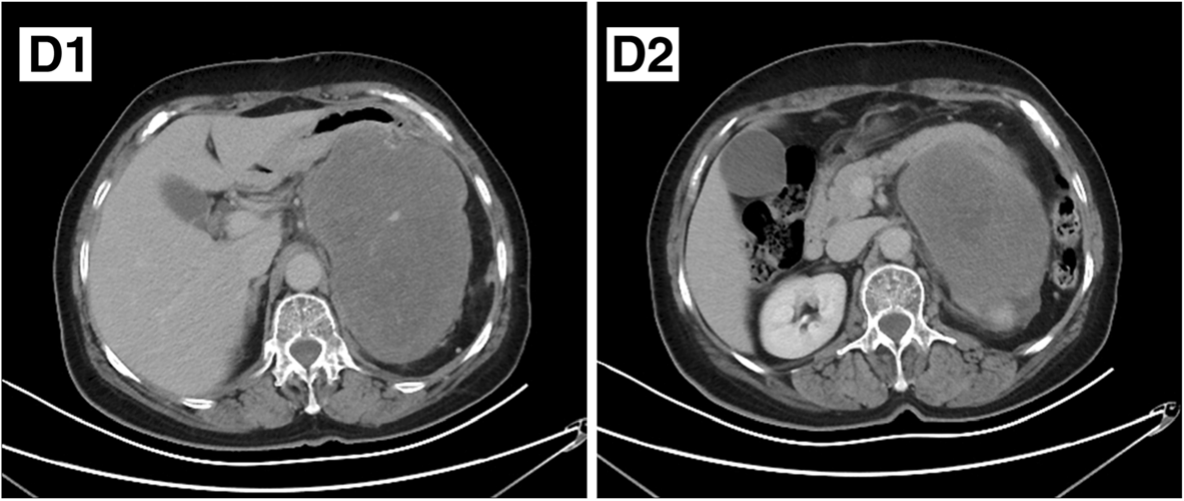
(D1) A huge, well-margined retroperitoneal MPNST is shown on enhanced CT scan, with feeding vessels inside. (D2) On the lower level, a hypo-attenuated foci can be observed, which was turned out to be a myxoid cyst
